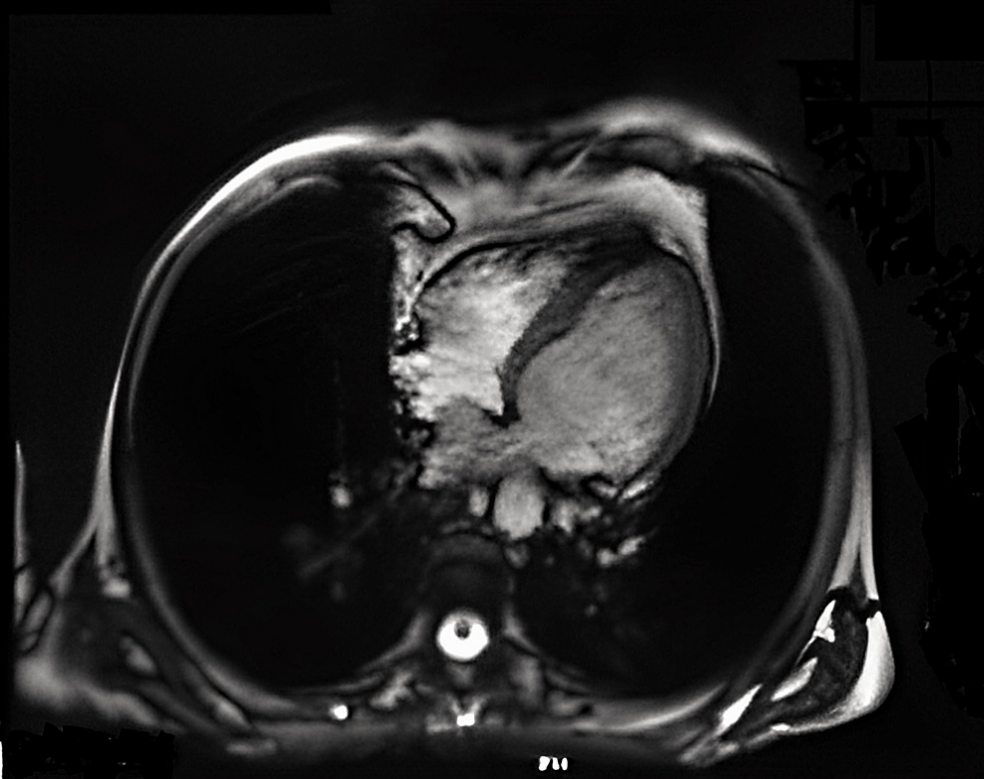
Cardiac MRI Cardiac function in recovery phase (ejection fraction of 45%).
